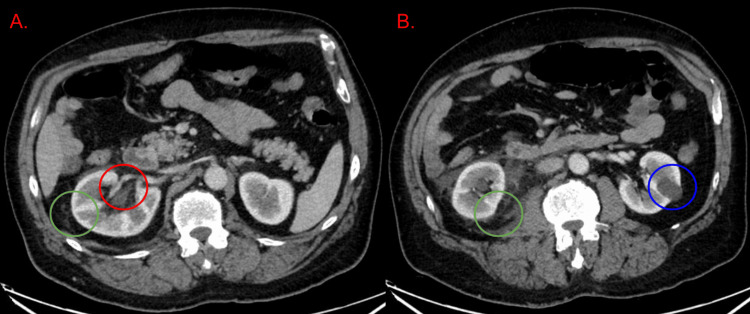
Transverse view from a computed tomography scan with contrast of the abdomen and pelvis A transverse view from a computed tomography scan with contrast of the abdomen and pelvis that depicts mild right-sided hydronephrosis and hydroureter (red circle) on panel A. It also shows right-sided perinephric stranding as well as a moderate amount of perinephric fluid (green circles) on panel A; these findings are nonspecific for forniceal rupture. A renal cyst (blue circle) can be seen on the left kidney on panel B.
